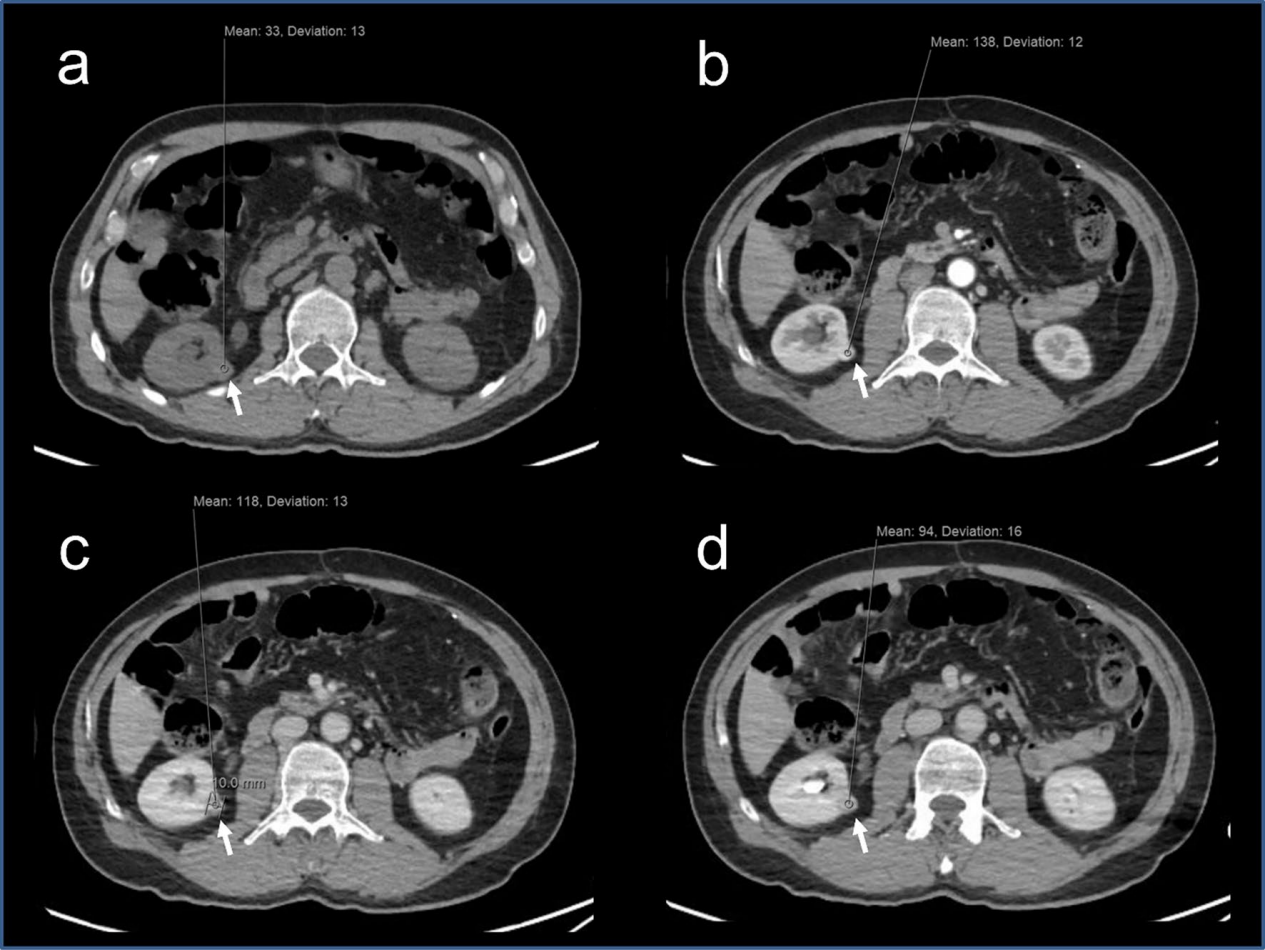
CT scan of the abdomen at follow up of the same patient after 2 years showing Hounsfield density measurement of the renal tumor consistent with right RCC (arrows). (a) CT without contrast, lesion density 33 ± 13. (b) Arterial phase, the density increased to 138 ± 12. (c) Venous phase the density decreased to 118 ± 13. (d) Delayed phase the density decreased to 94 ± 16.
